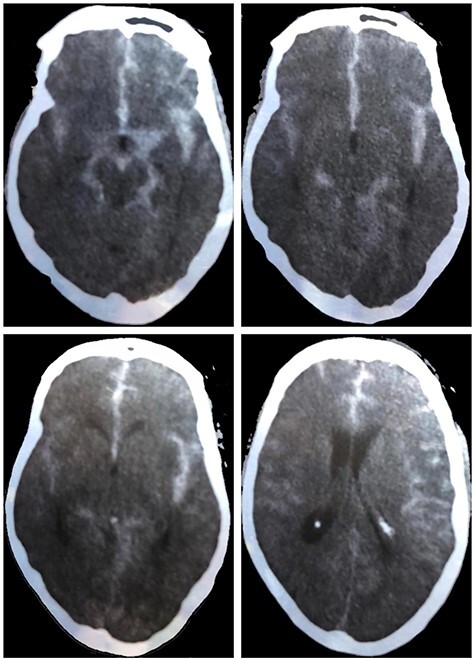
Unenhanced CT scan at the time of presentation demonstrating diffuse SAH with the main brunt of hemorrhage in the left suprasellar cistern and Sylvian fissure.
